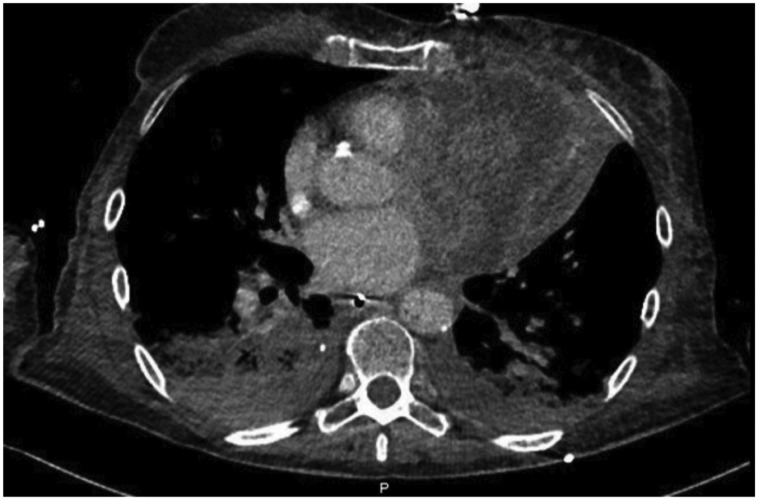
Thoracic computed tomography scan showing a heterogeneous mass, with necrotic material, originating from the inferior left lung lobe invading the anterior and lateral wall of the left ventricle, left branch of the pulmonary artery, left bronchi and anterior chest wall.
